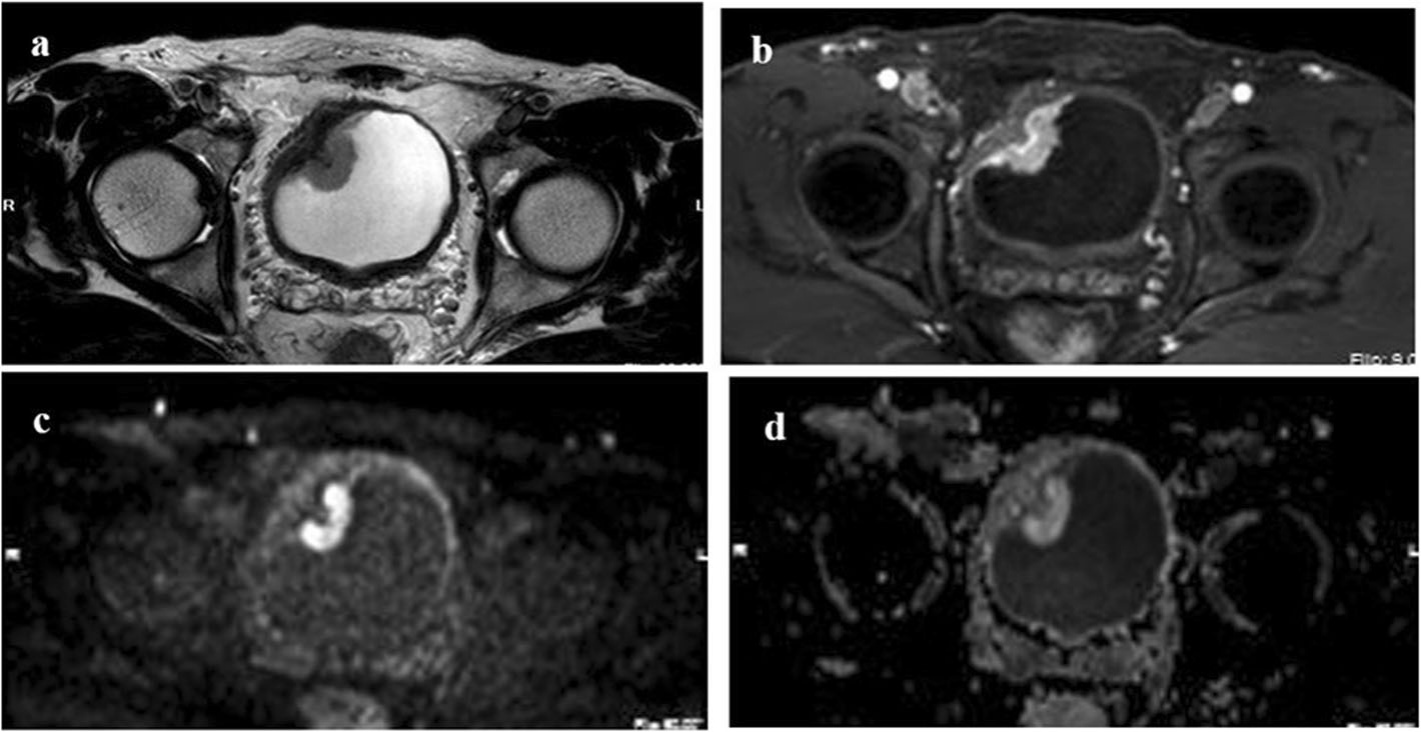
Image sequences of a single tumor with a score of VI-RADS 2 on the transverse plane. (a): T2-weighted imaging of tumors with a score of VI-RADS 2 on transverse plane; (b): Dynamic contrast enhanced imaging on transverse plane; (c)and(d): diffusion-weighted imaging on transverse plane
